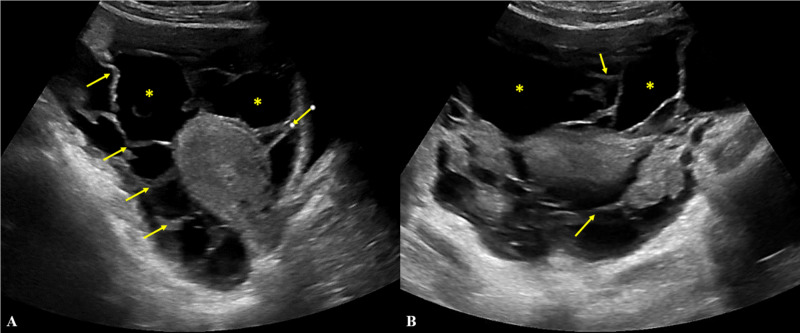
Transabdominal pelvic ultrasound with selected sagittal (A) and axial (B) views of the uterus This study was significant for “complex septated fluid throughout the pelvis”. Free fluid is identified by asterisks. Septations identified by arrows
